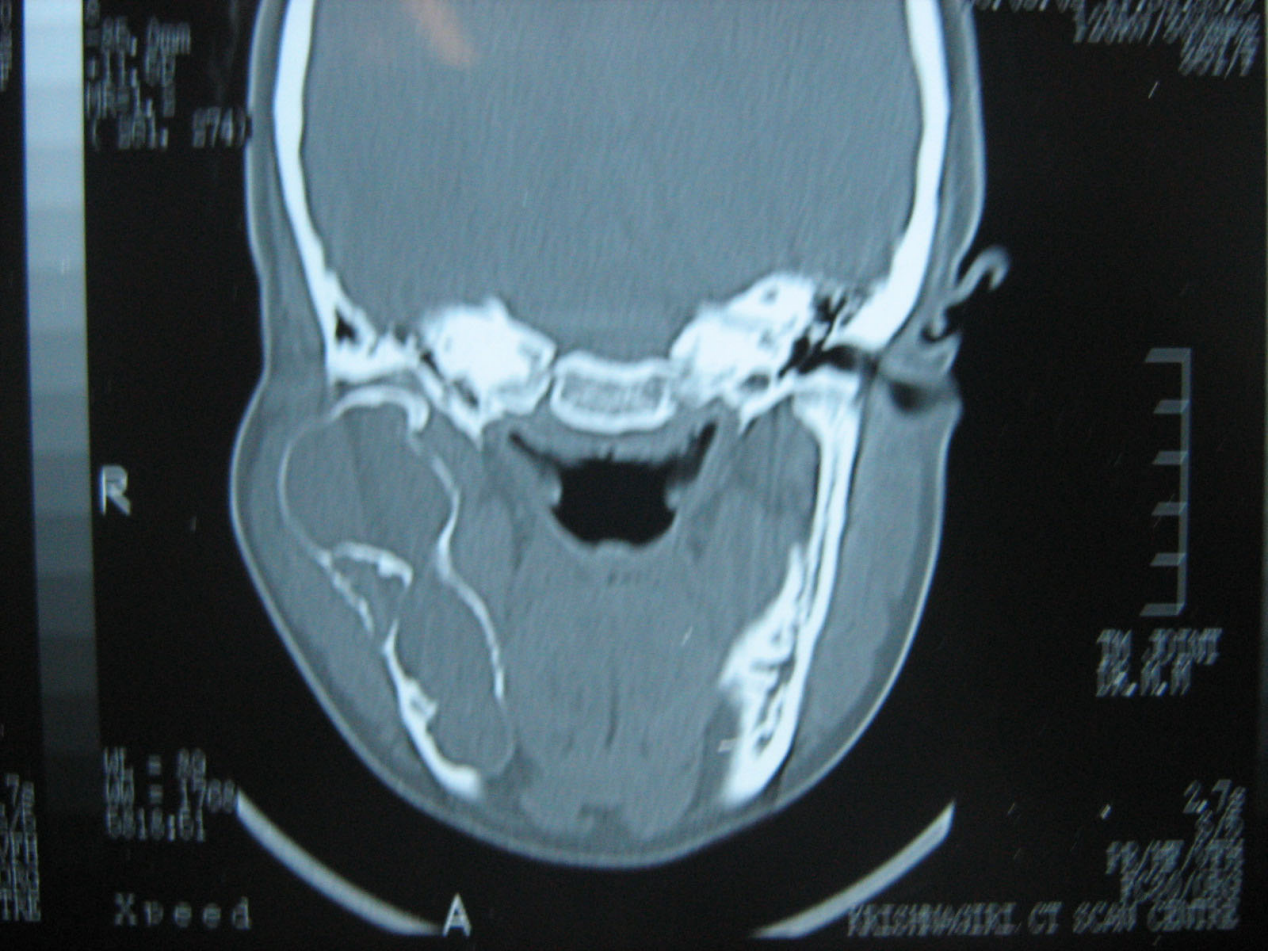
CT scan showing lesion in right hemimandible.
